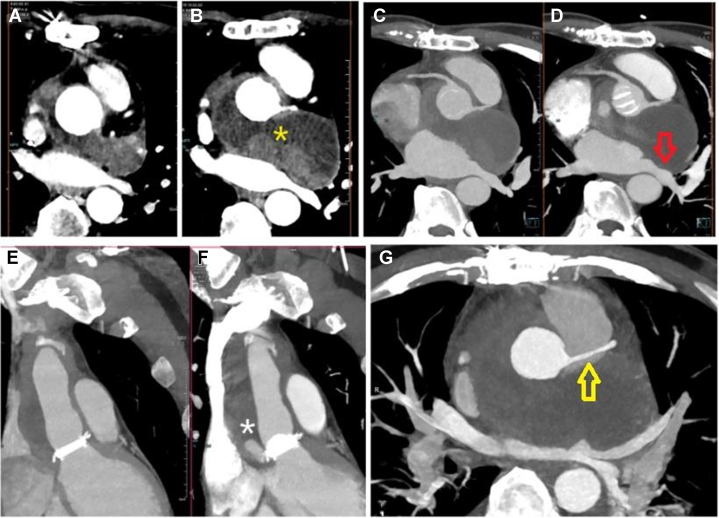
Findings on Computed Tomography Contrast-enhanced cardiac CT showing marked and rapid expansion of the perigraft fluid collection surrounding the ascending aortic conduit (A and B). Multiplanar reconstructions demonstrate focal dehiscence at the proximal suture line of the valved conduit with contrast extension toward the perigraft space, consistent with an anastomotic leak (E and F). The enlarging collection exerts mass effect on adjacent structures, producing extrinsic compression of the left superior pulmonary vein (red arrow) followed by significant narrowing of the right pulmonary artery (yellow arrow) (C, D, and G). (B) The yellow asterisk denotes the perigraft fluid collection. (F) The white asterisk denotes the focal dehiscence at the proximal suture line of the valved conduit. CT = computed tomography.
